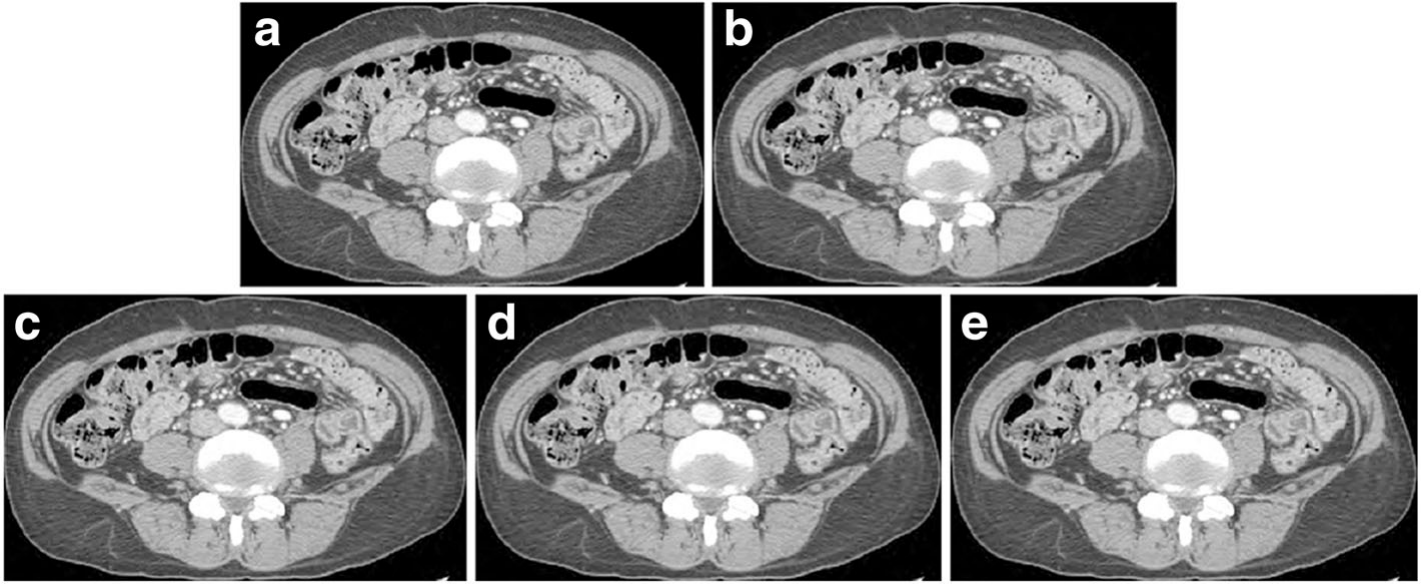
JPEG 2000 compressed CT images in transverse abdominal view of a 78-year-old female. (a) the original image. (b) the compressed image by a radiologist. (compression ratio 8.6:1.) The compressed images using (c) the MLR model (8.8,1), (d) the PSNR (9.5,1), and (e) the HDRVDP (9.3,1) respectively. Window width and level are set to 400 and 20 HU. Since the images are generated by visually lossless compression methods, all the images are indistinguishable from the original image
